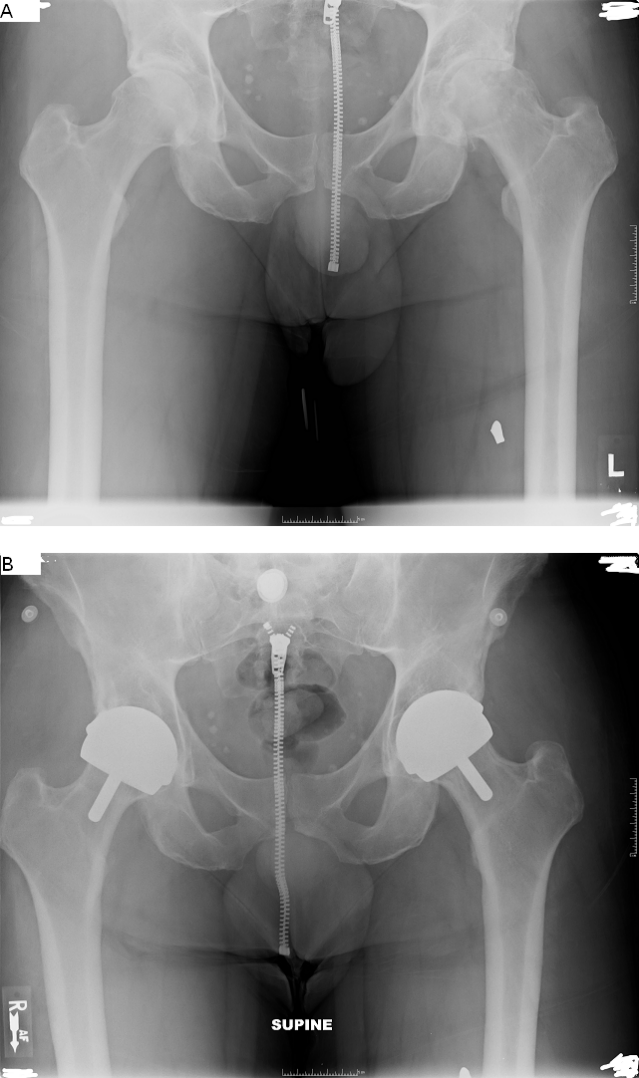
Bilateral HRAs, male 43 years old age, the cyst size of 3 cm^3 ^on the left side and the cyst size of 0 cm^3 ^on the right side; HHS 97 at both 3-year follow-up (left) and 1-year follow-up (right), primary diagnosis of OA for both side. A: pre-operative x-ray, B: latest post-operative xray.
